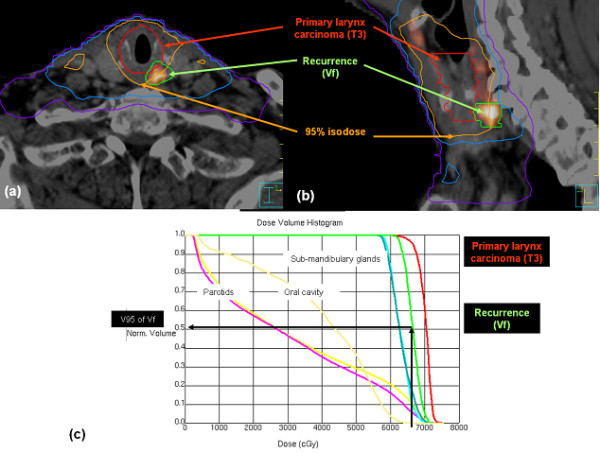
Example of marginal recurrence. The planning CT has been registered with the FDG-PET-CT imaging of the recurrence. The failure volume (*Vf) *appears partially outside the 95% PTV isodose (a and b). The *Vf*-V95 is equal to 50% (c).
